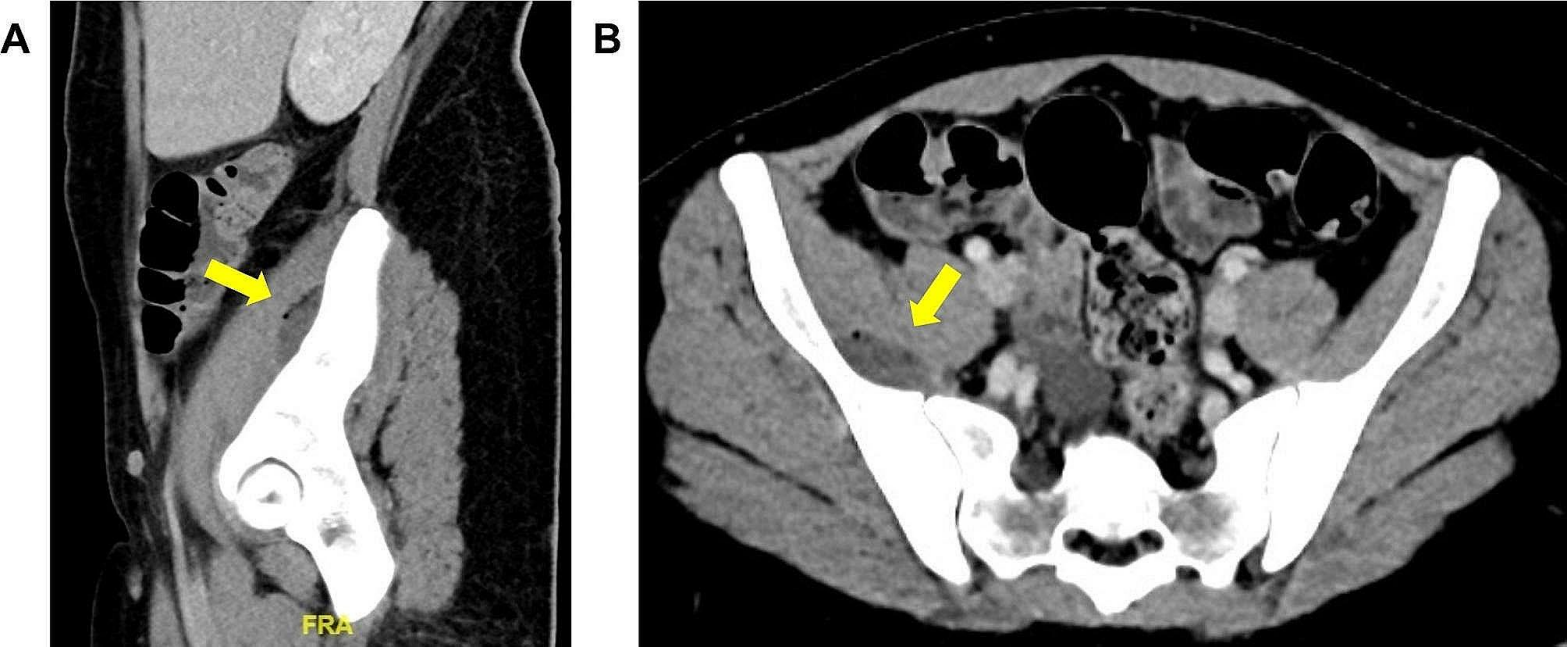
Computed tomography imaging with contrast, (A) sagittal and (B) axial views. Arrow: a gas-containing, faintly rim-enhancing fluid collection (17 ± 8 Hounsfield unit) at the deep aspect of the right iliacus muscle overlying the right sacroiliac (SI) joint measuring 3.4 × 1.2 × 3.9 cm
